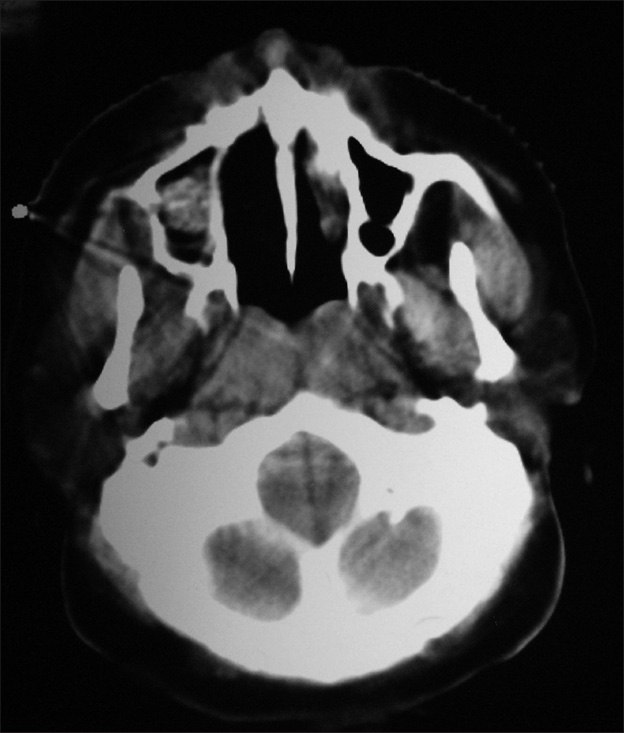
Axial CT scan showing tumor in the right maxillary sinus
